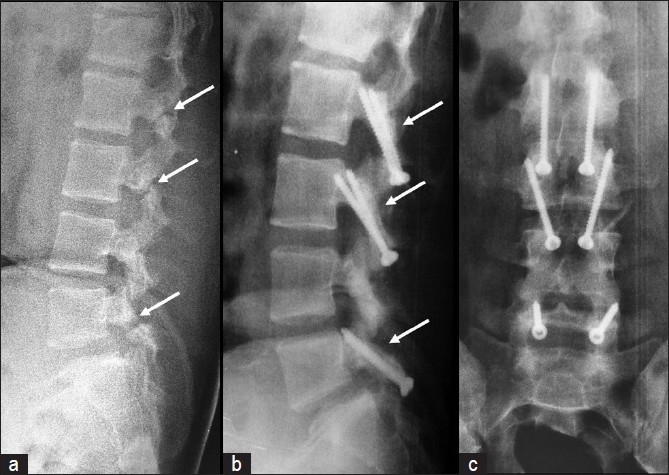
(a) Preoperative lateral radiograph of a patient with triple level spondylolysis at L2, L3, and L5. (b) Postoperative follow-up lateral and (c) anteroposterior radiographs showing good healing of the pars defect at all three levels
